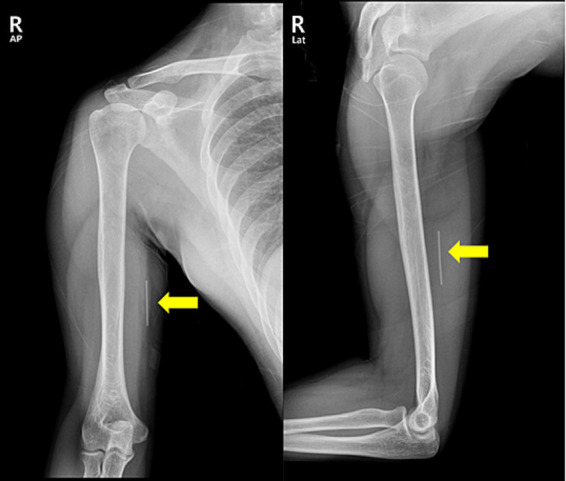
X-ray image of the etonogestrel implant (yellow arrow) positioned in the right upper arm of a 33-year-old woman (Busan, South Korea, 2025).
